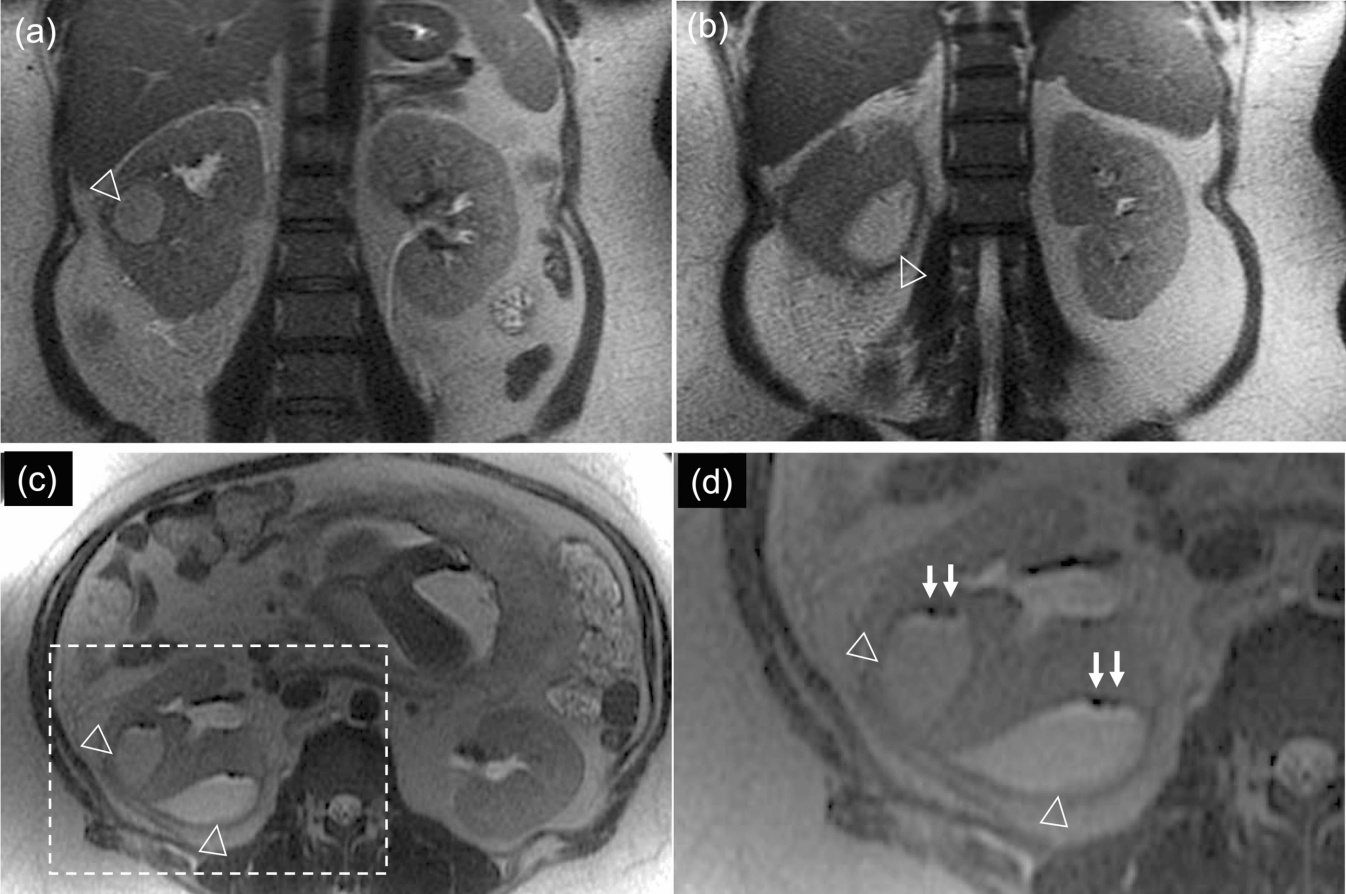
T2-weighted magnetic resonance images at 30 weeks of gestation Multiple renal abscesses (arrows) in the right kidney showed hyperintensity in the coronal (a,b) and transverse (c,d: magnified view of the dotted square area) views, while air within the abscesses (arrowheads) appeared hypointense (d).
